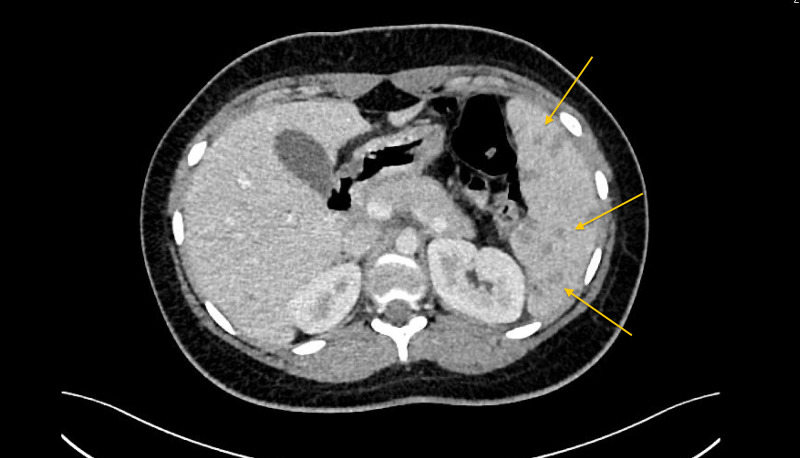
CT scan of the abdomen showing multiple hypodense lesions in the spleen (marked by yellow arrows)
